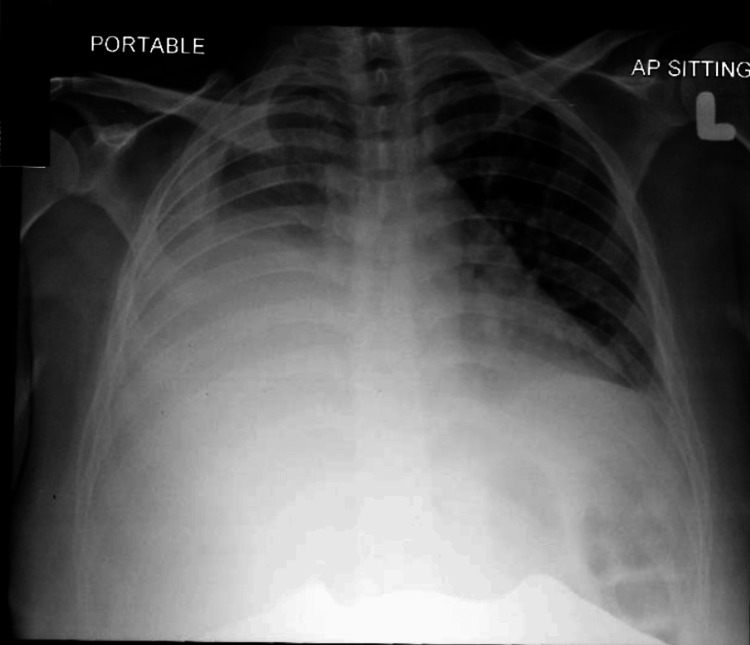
The chest X-ray which showed massive right-sided pleural effusion
